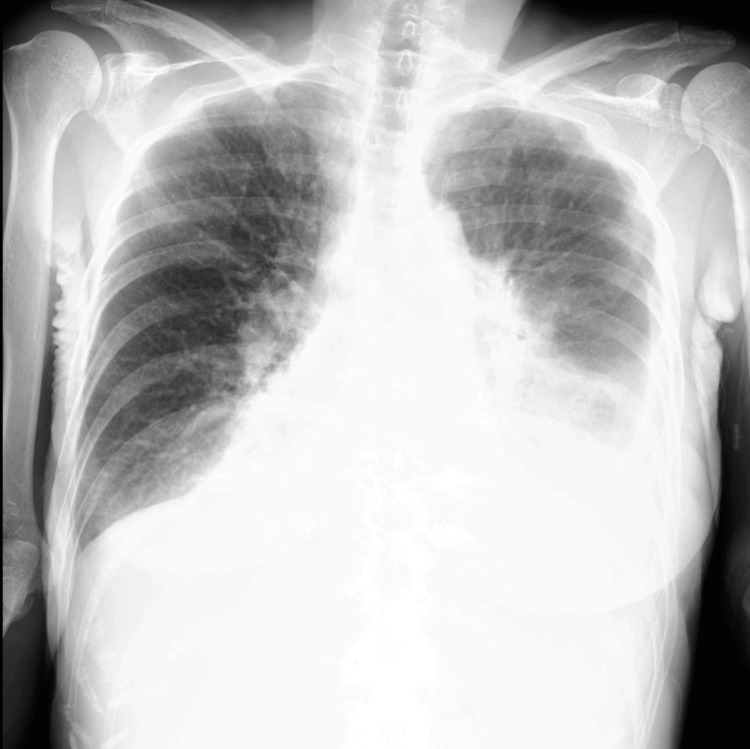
X-ray of the first visit X-ray showing obvious cardiac enlargement and pulmonary congestion because of chronic heart failure
